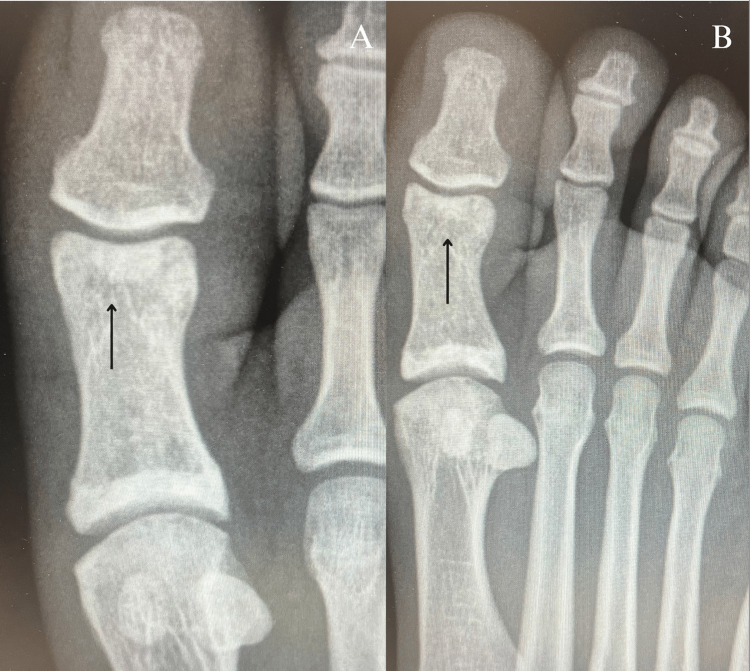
Plain anteroposterior (A) and oblique radiographs (B) of the right foot taken nine weeks and three days from the initial injury show complete ossification of the sesamoid bone fracture at the distal interphalangeal joint of the great toe (black arrows)
